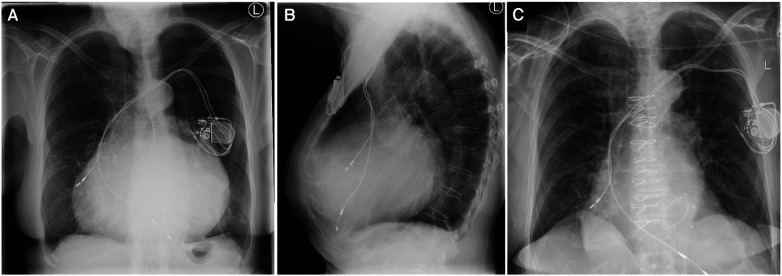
(A) Chest x-ray prior to surgery, (B) lateral chest x-ray prior to surgery, and (C) chest x-ray after surgery.
